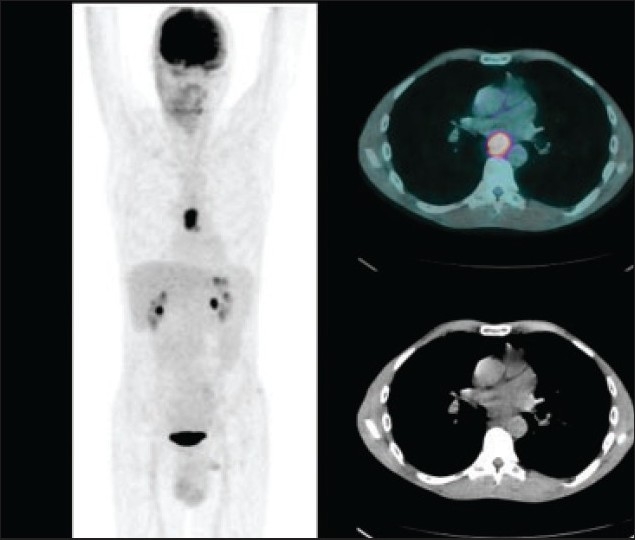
A 56-year-old man who came for initial staging of esophageal cancer. The MIP (left panel) and axial fused (right upper panel) and axial CT (right lower panel) images of 18F-FDG PET/CT showed the primary mid-esophageal tumor with no evidence of FDG-avid distant metastases.
